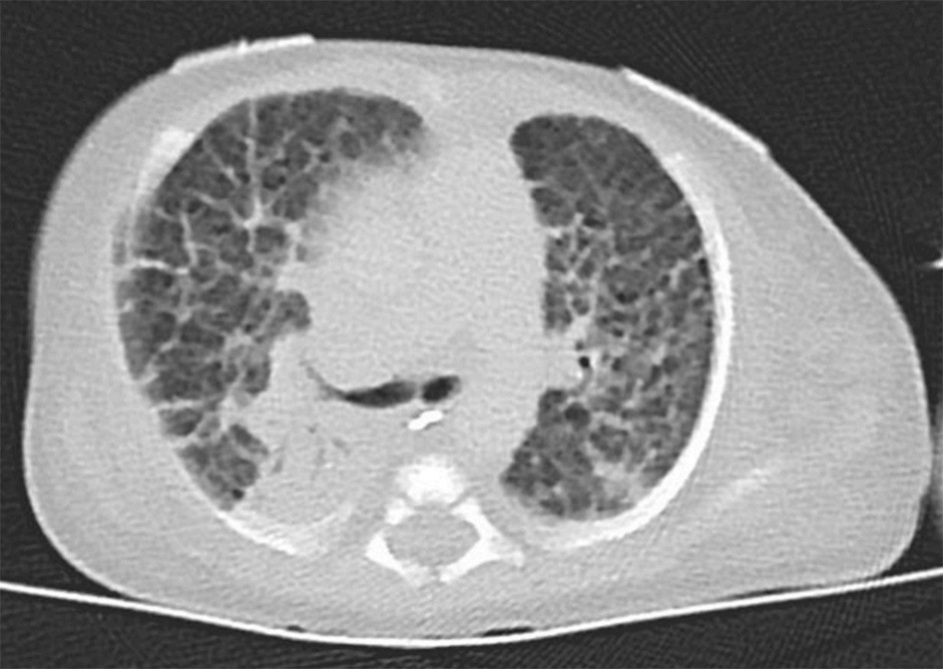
Thoracal CT-scan at 7 weeks showed an interstitial opacity indicating a chronic pulmonary disease.
